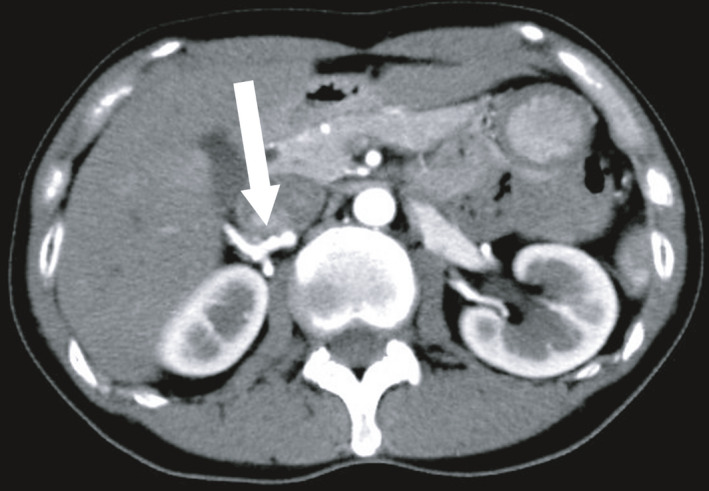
Contrast‐enhanced CT showing irregular lumen of the right renal artery (arrow).
